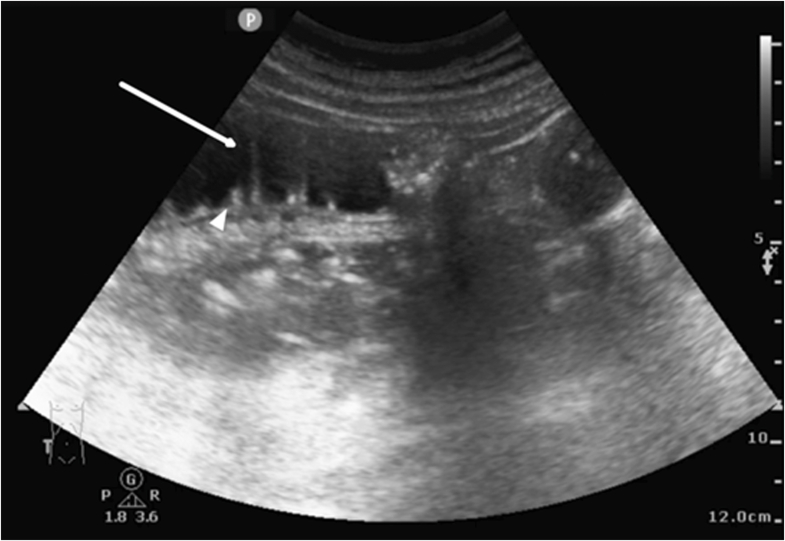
Point-of-care ultrasound demonstrating prominent plicae circulares (arrow) within a fluid-filled small bowel loop, known as keyboard sign (arrowhead).
